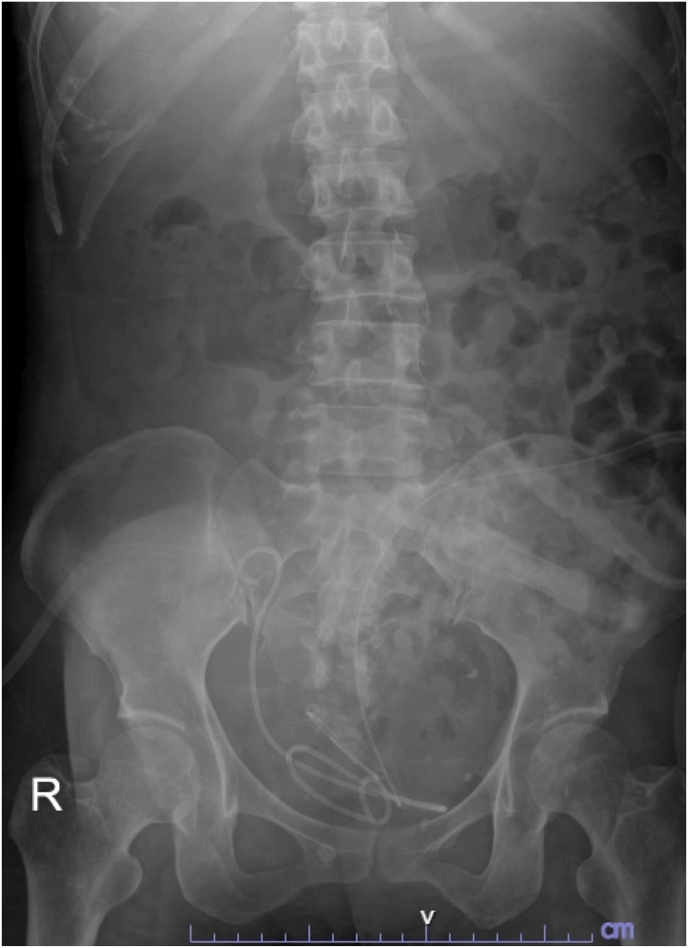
Postoperative abdominal radiography.
